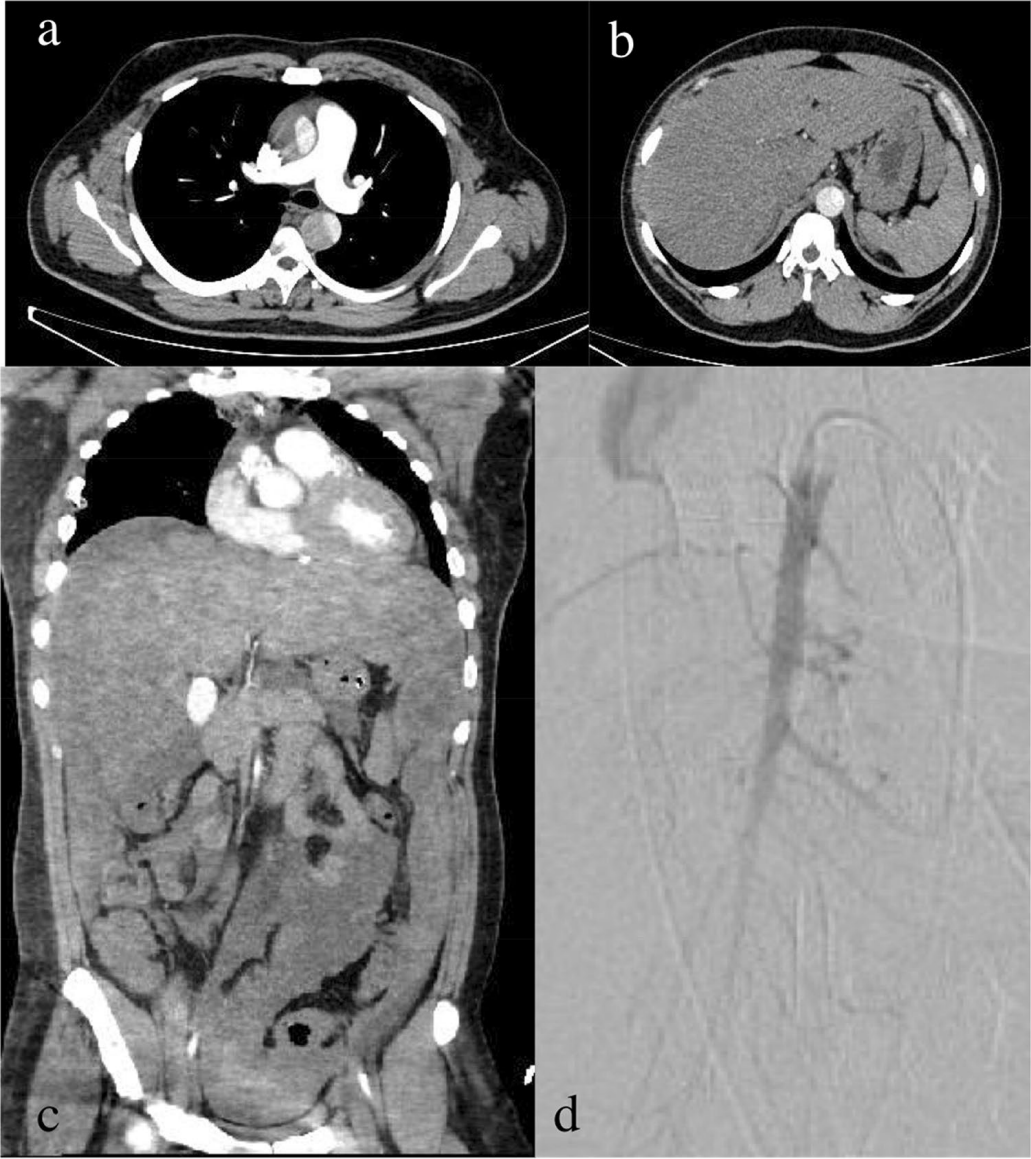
a, b Contrast-enhanced computed tomography (CT) showing type A aortic dissection and splenomegaly. c CT after the first operation showing ischemic small intestine showing a reduced contrast effect in peripheral region of superior mesenteric artery (SMA) and maintained contrast effect in the main trunk of SMA, along with the spleen and the liver infarct. d Angiography image showing reduced vessel filling in the peripheral region of the superior mesenteric artery
